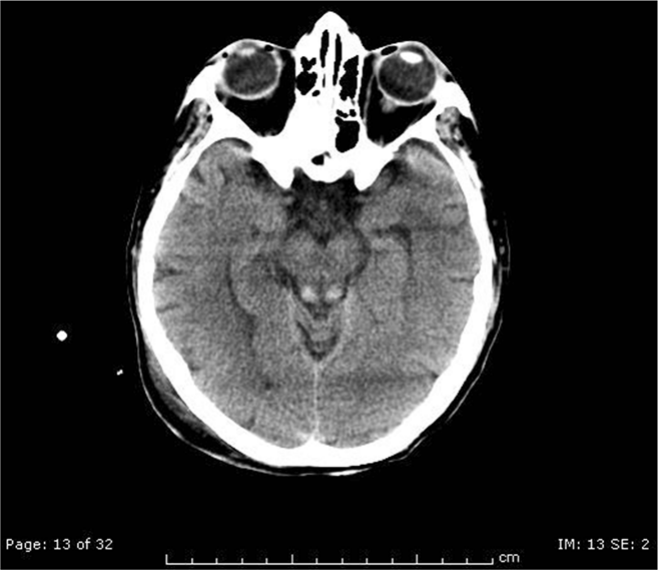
CT scan shows faded hyperdensity of the mammillary bodies and symmetrical hemorrhagic lesions in the quadrigeminal plate.
